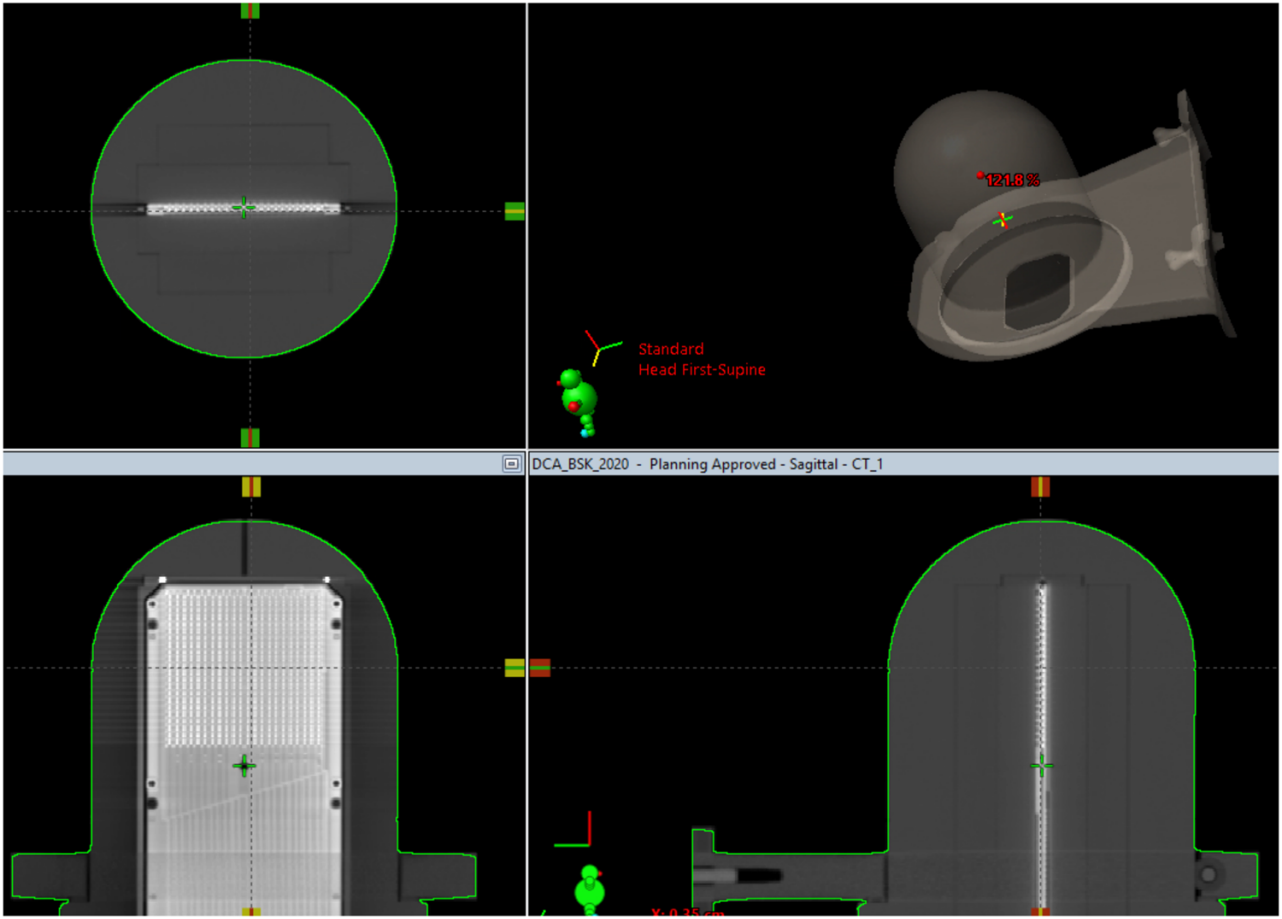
Three orthogonal views of CT imaging of the StereoPHAN/stereotactic radiosurgery (SRS) MapCHECK
